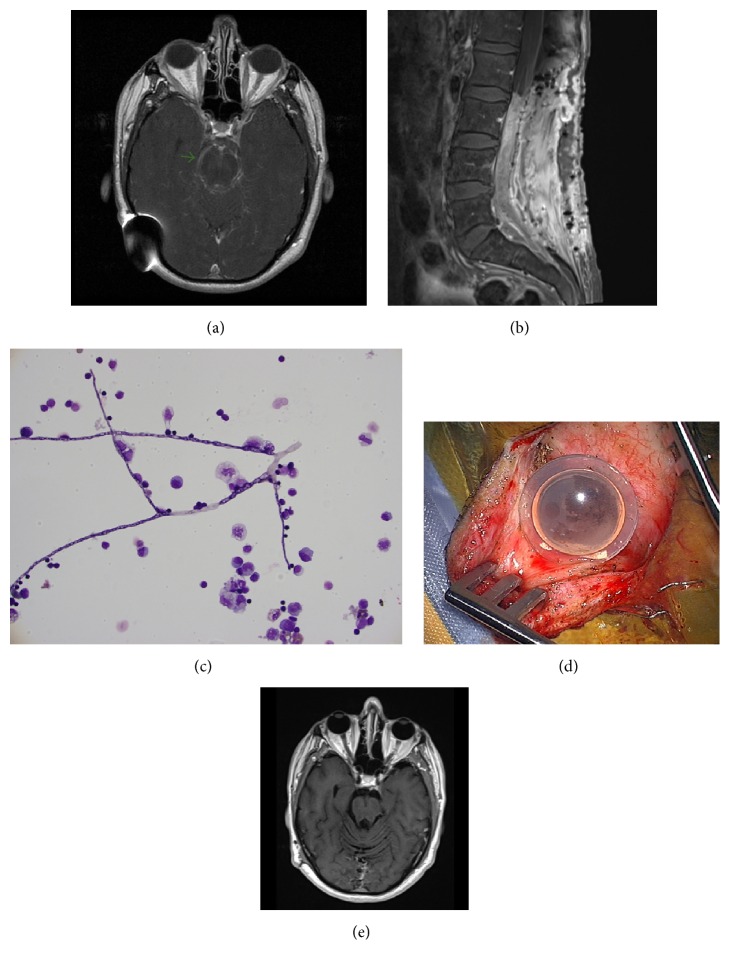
((a) & (b)) Pretreatment MRI images, (c) CSF cytology showing hyphae and inflammatory response, (d) discolored Ommaya reservoir, and (e) posttreatment MRI.
